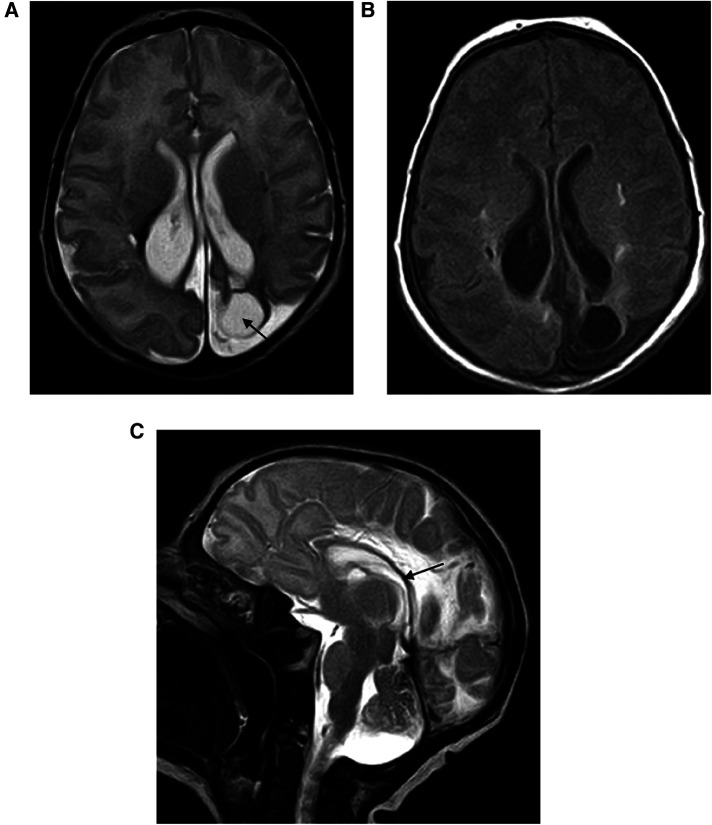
(A,B) Encephalomalacia secondary to parenchymal hemorrhage: axial T2-weighted image shows the limited area of parietal encephalomalacia (black arrow) with *ex vacuo* enlargement of the lateral ventricle. (C) Corpus callosum thickness reduction (black arrow).
